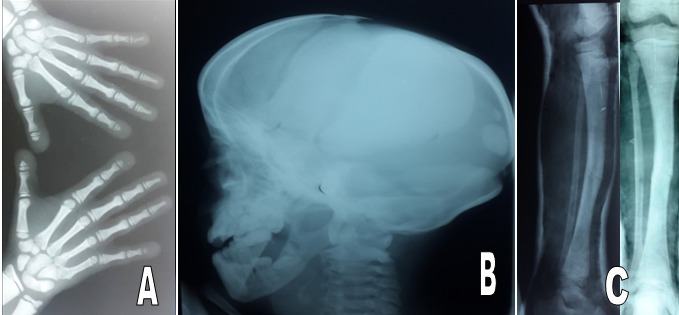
A) AP X-ray of both hands demonstrate short stubby fingers with dysplastic nails and acro-osteolysis; B) lateral skull X-rays reveal a large cranial vault with persistent open sutures and fontanelles as well as wormian bones: hypoplastic facial bones and an underdeveloped mandible with an obtuse jaw angle are also demonstrated; C) AP and lateral X-ray reveal a tibial shaft fracture
